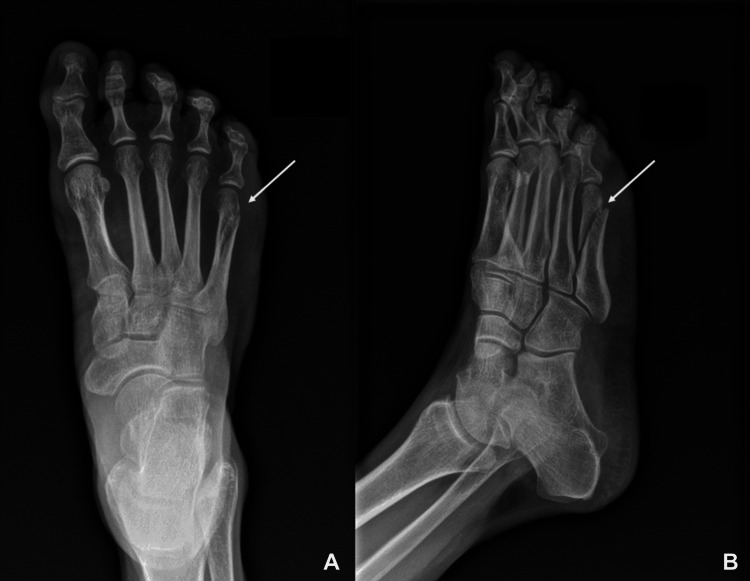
X-ray images of the fifth metatarsal fracture (A) Anteroposterior view; (B) oblique view
